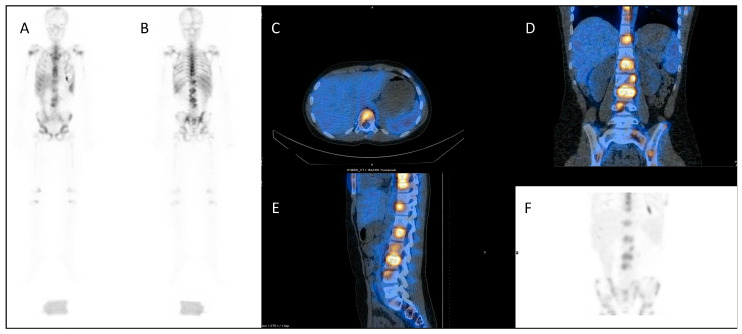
Dosimetry imaging with ^111^In-labelled anti-CD66 monoclonal antibody in a patient with relapsed leukaemia prior to ^90^Y anti-CD66 monoclonal therapy in an early phase trial [50]. (A) Anterior and (B) posterior whole-body planar scintigraphy views; (C) axial, (D) coronal and (E) sagittal fused SPECT CT views; and (F) SPECT maximum intensity projection image. These demonstrate avid uptake in areas of involved bone marrow.
